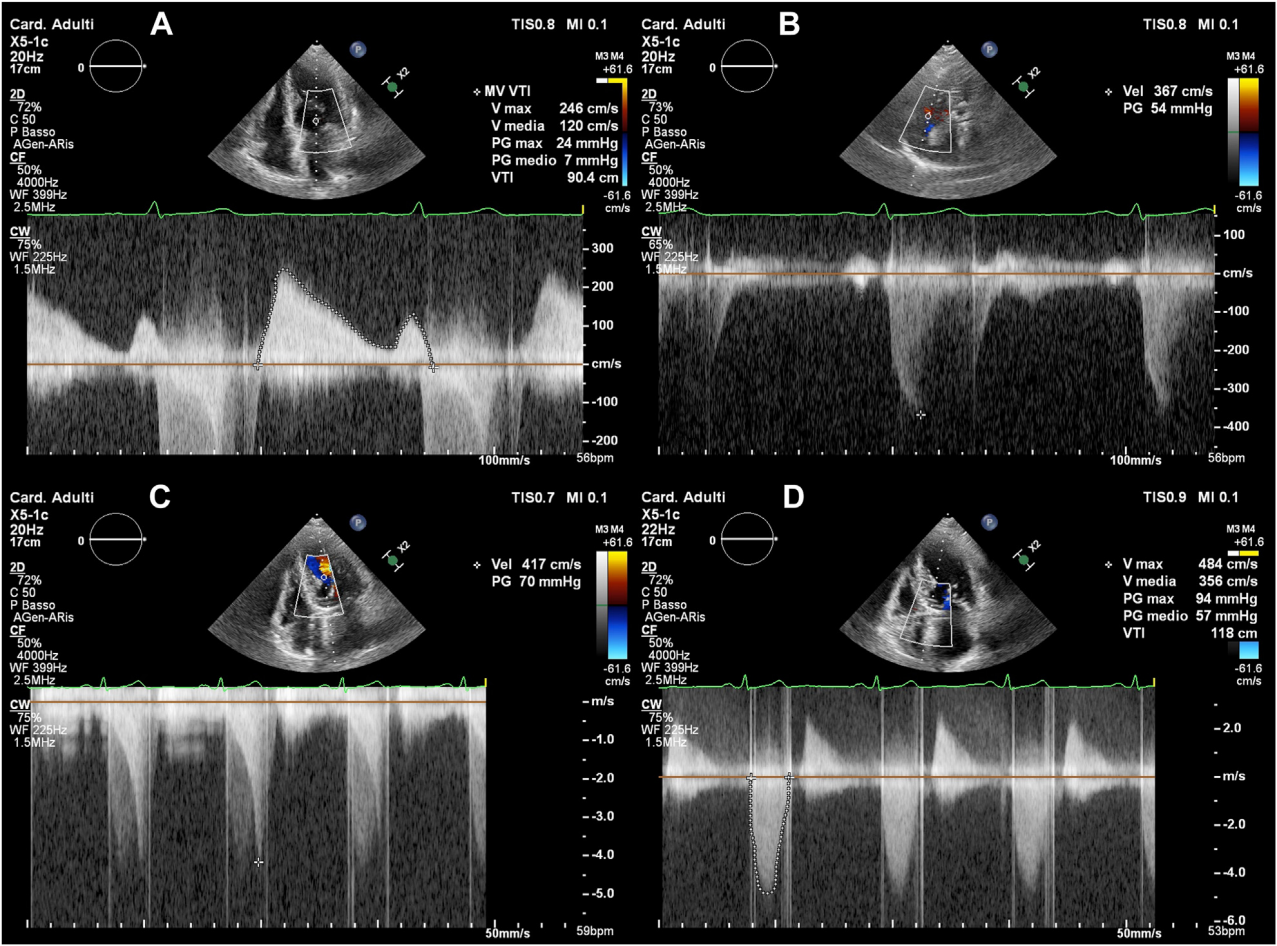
Two-dimensional TTE-guided continuous-wave Doppler spectral profiles from the apical 4-chamber view demonstrate (A) the diastolic mean gradient (7 mm Hg) across the MV; (B) the maximal tricuspid regurgitation velocity (367 cm/sec) and estimated gradient (54 mm Hg); (C) the “dagger-shaped” midventricular functional systolic resting gradient (70 mm Hg) across the LV outflow tract; and (D) the peak (94 mm Hg) and mean (57 mm Hg) fixed systolic gradients across the aortic prosthesis.
